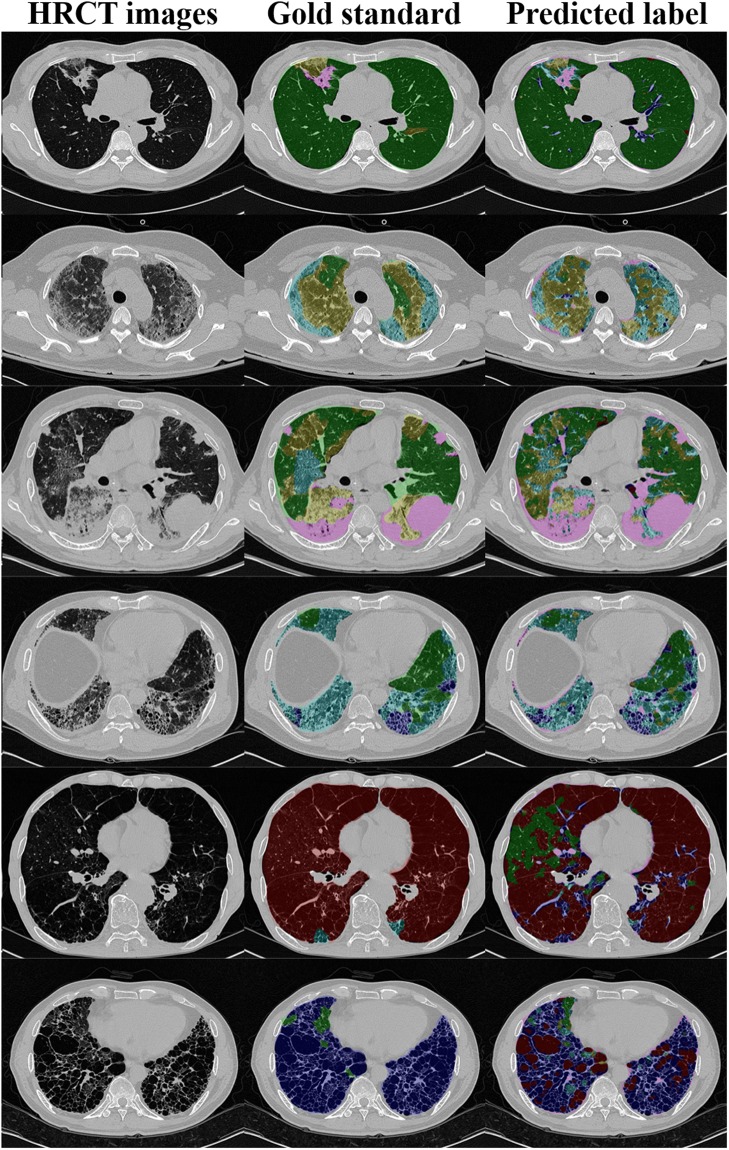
Examples of 2D HRCT images (left) with the corresponding gold-standard classifications of radiologist 2 (middle) and the CNN predicted labels (right). From top to bottom, the dominant patterns of DILD are normal (green), GGO (yellow), consolidation (pink), RO (cyan), emphysema (brown), and honeycombing (blue).
